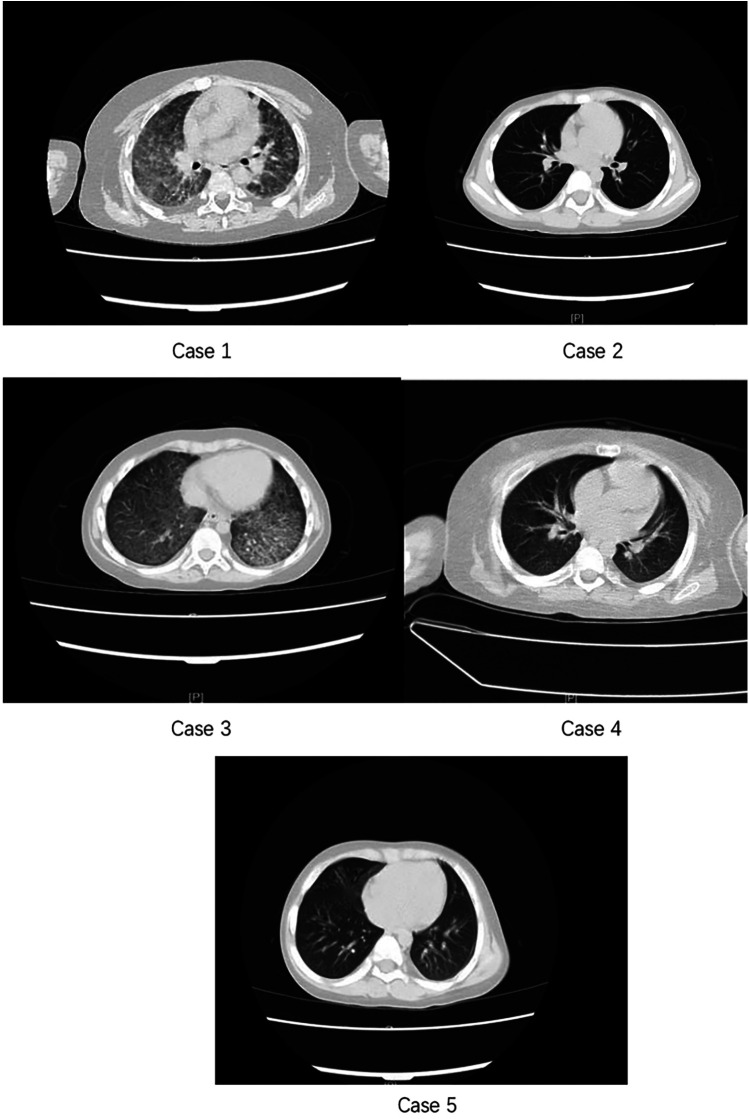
Chest computed tomography scans of five children with nephrotic syndrome complicated by Pneumocystis jiroveci pneumonia (Cases 1–5). The images show bilateral interstitial and alveolar infiltrates of varying extent, with some cases demonstrating pleural effusion and more extensive parenchymal involvement.
